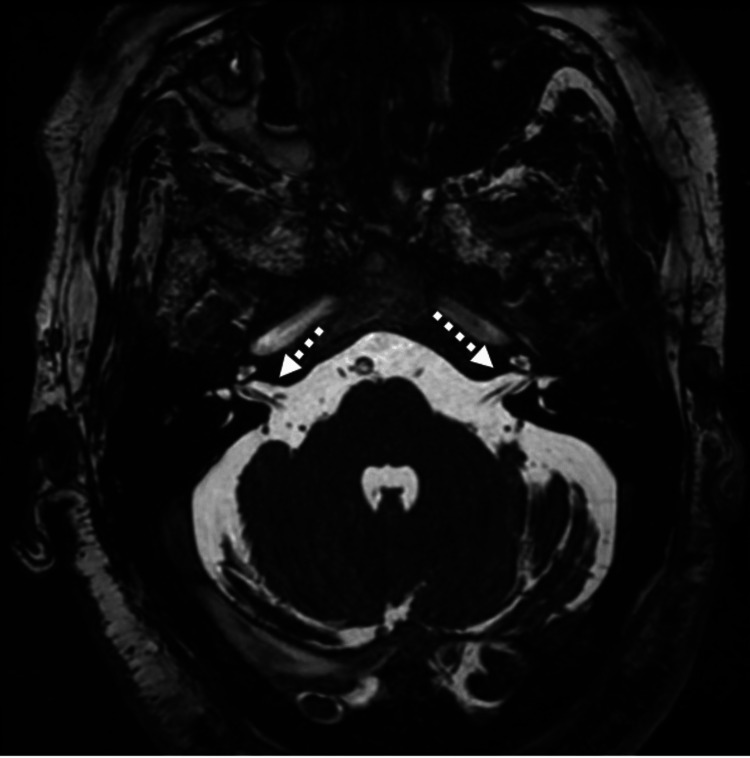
MRI of the temporal bones Axial 3D FIESTA MRI demonstrates normal bilateral internal auditory canals and inner ear structures (dashed white arrows), with no abnormal enhancement or pathological findings.
